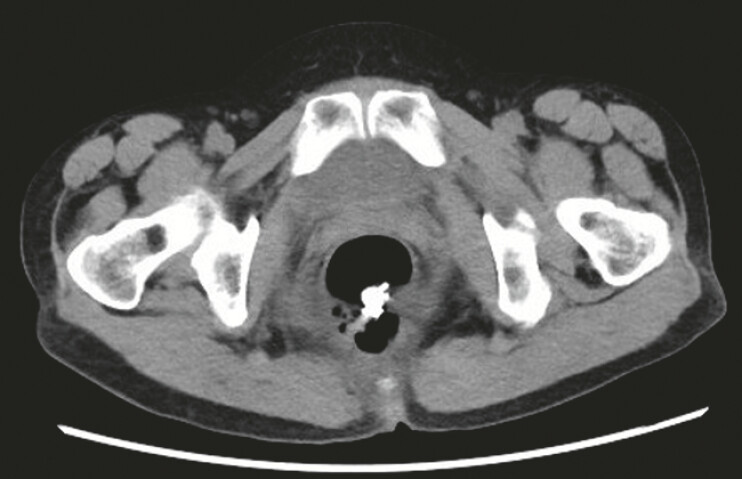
A postoperative CT scan confirming the complete excision of the cyst. CT, computed tomography.
